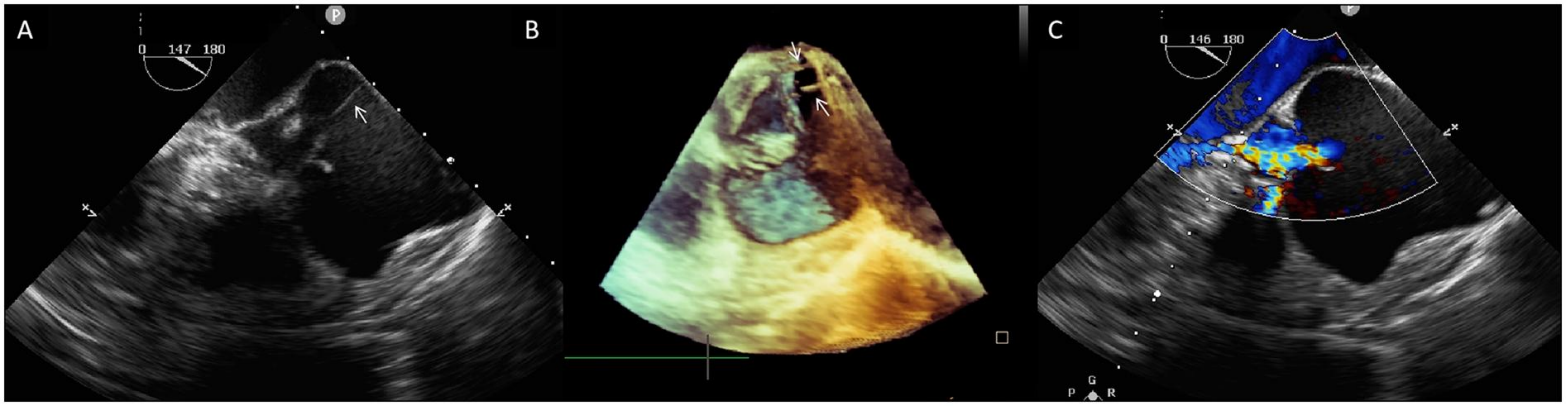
(A) Two-dimensional transesophageal echocardiography (TEE) shows a fibrous strand (arrow) extending from the aortic sinus wall to the free rim of the non-coronary aortic cusp. (B) Three-dimensional TEE shows two aortic chordae tendineae (white arrows)attaching the non-coronary cusp to the aortic sinus wall. (C) Color Doppler TEE demonstrates severe central aortic regurgitation (vena contracta: 7 mm).
